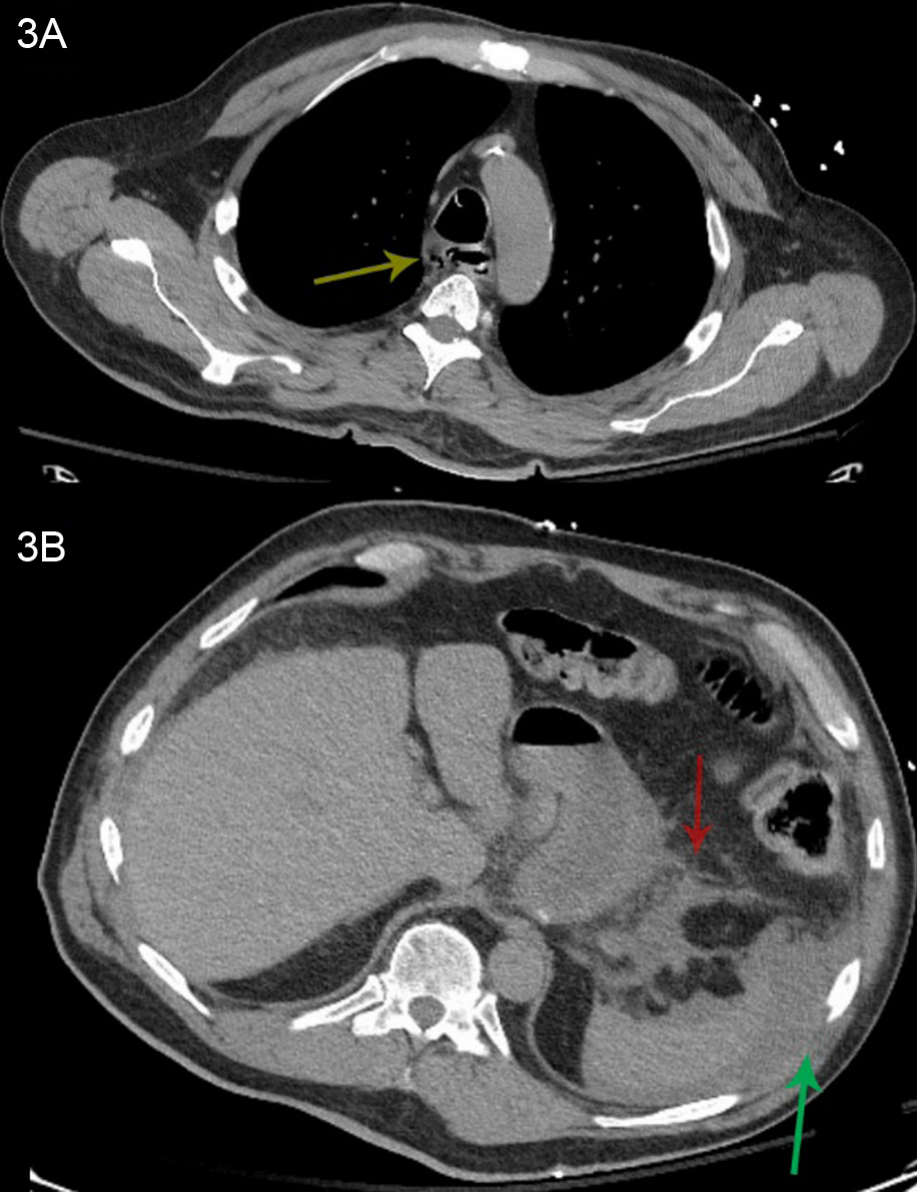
Computed tomography (CT) of the chest and abdomen one day later. 3A: CT chest without contrast, same region cut as 1A. The yellow arrow indicates mediastinal air around the esophagus. 3B: CT abdomen pelvis without contrast, same region as 1B. The red arrow indicates free fluid around the stomach; the green arrow indicates free fluid around the spleen.
